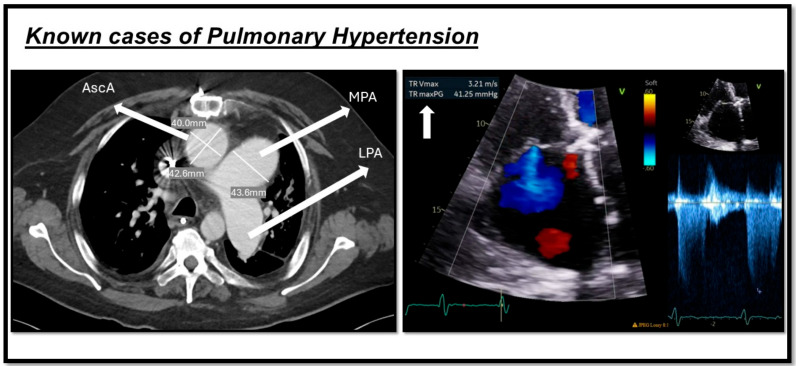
Shows two cases of pulmonary hypertension. The image on the left indicates a CT scan of one of our patients, demonstrating a dilated main pulmonary artery (MPA) measuring more than 29 mm, along with an increased MPA-to-aorta ratio (>1). The image on the right depicts echocardiography from another patient with pulmonary hypertension, showing an increase in tricuspid regurgitation velocity (TRV) as a marker of the condition. This figure is presented as part of an educational poster session at Radiology Society of North America (RSNA 2024). There is no copyright issue. 2024, Shafeghat. M.
